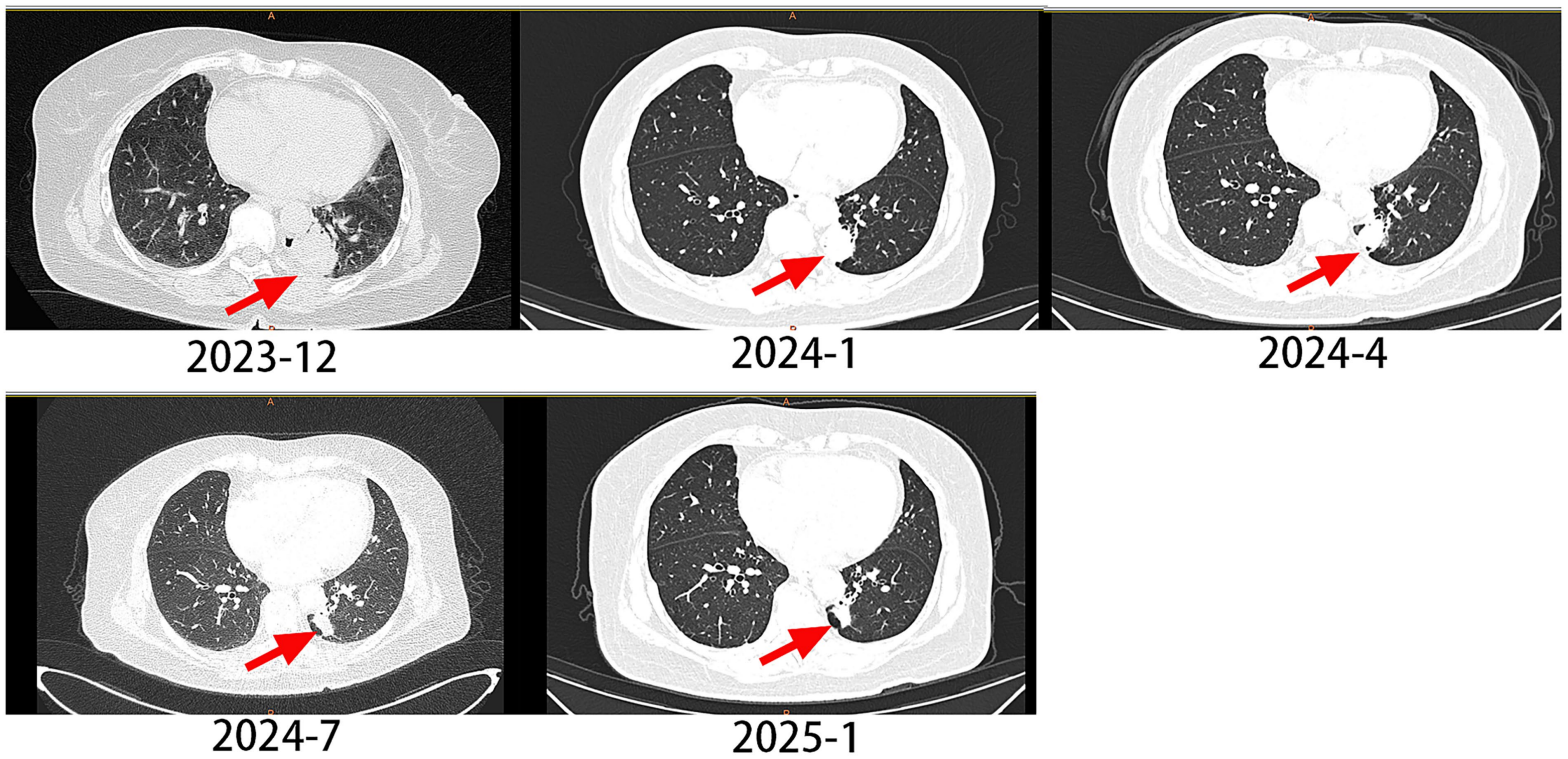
The changes of lung CT scan in case 1.
